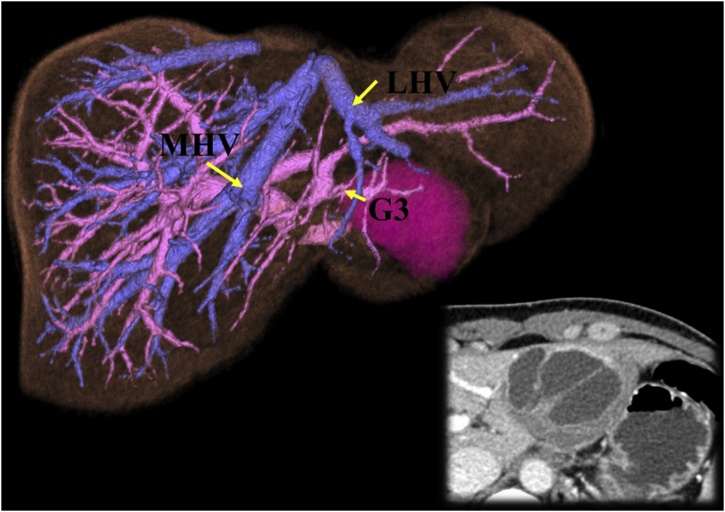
The three-dimensional imaging based on computed tomography showed mucinous cystic neoplasm, located at the segment III in the form of compressing the root of the Glissonean pedicle of segment III. MHV, middle hepatic vein; LHV, left hepatic vein; and G3, Glissonean pedicle of segment III.
